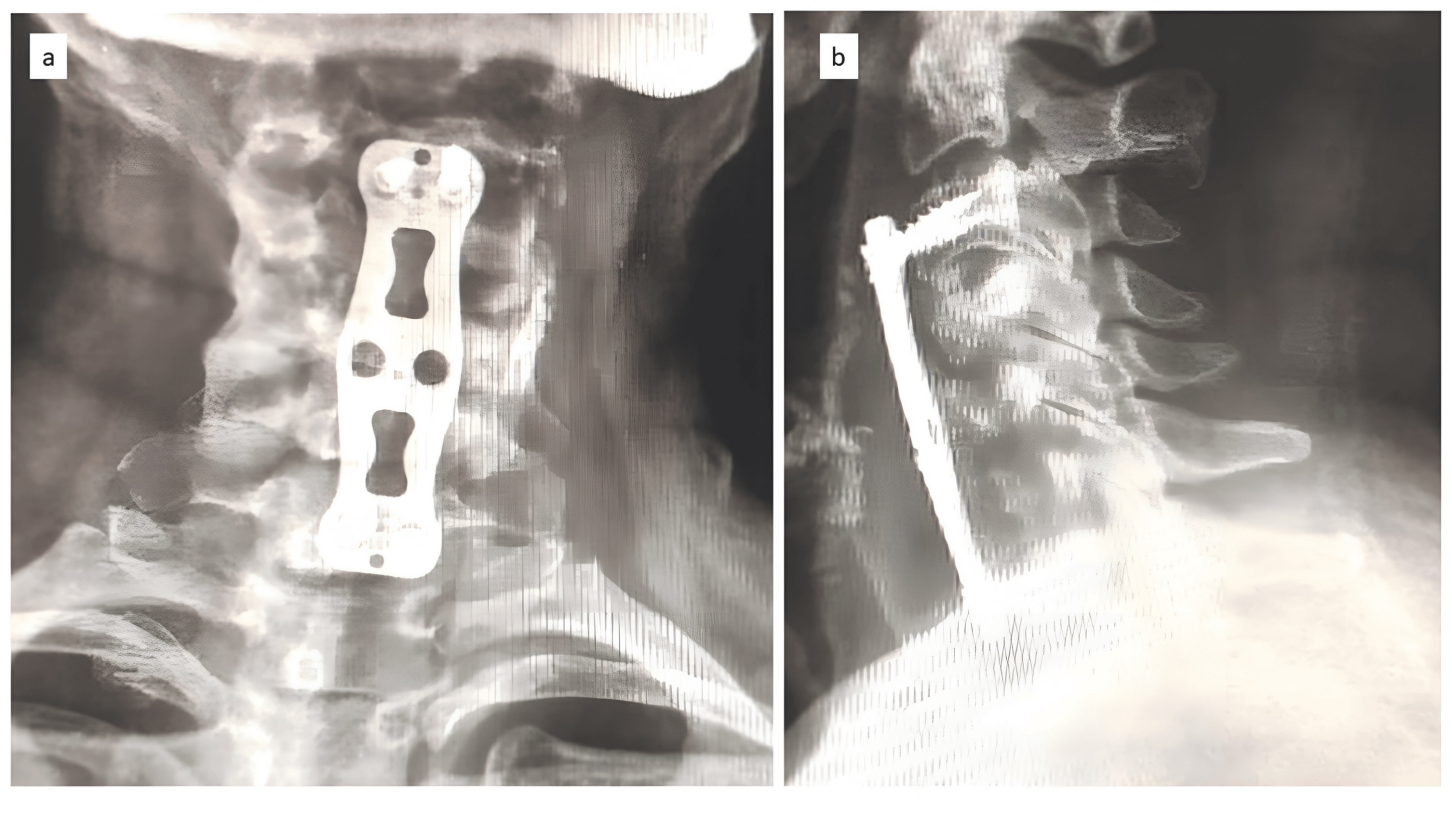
Postoperative radiographs after revision surgery showing extension of the fusion from C3 to C7. The patient underwent repair of the esophageal perforation and long-segment fusion from C3 to C7; (a) anterolateral postoperative radiograph and (b) lateral postoperative radiograph
